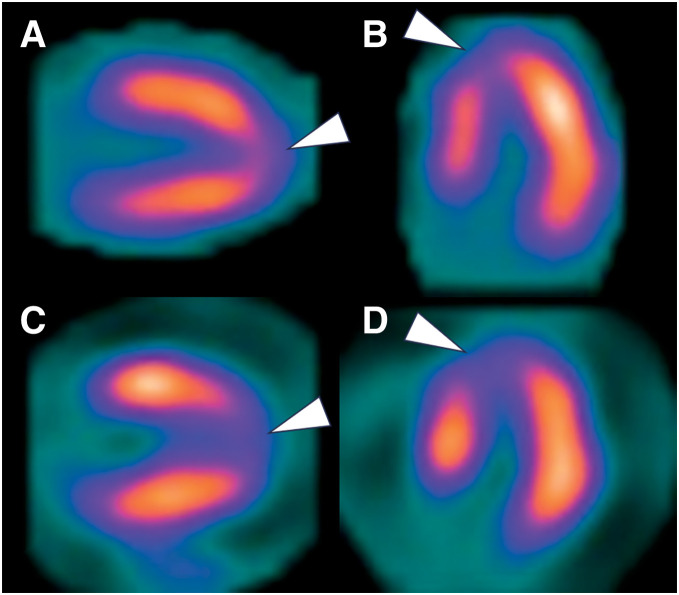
Myocardial scintigraphy findings. Myocardial scintigraphy with Thallium-201 (A, B) and I-123 BMIPP (C, D) revealed an apical defect (arrowheads), with the defect being more pronounced in the I-123 BMIPP images. I-123 BMIPP, Iodine-123 beta-methyl-p-iodophenyl-pentadecanoic acid
